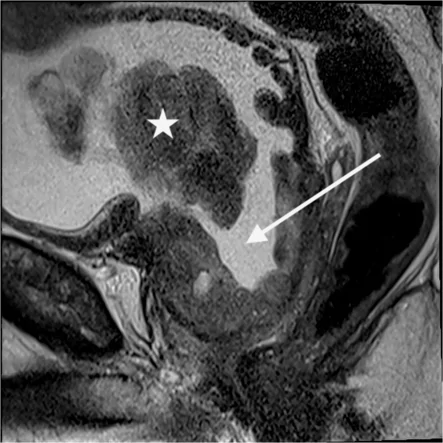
MRI Prostate performed on a different patient 6 weeks after PAE. A new prostatic defect is present at the bladder neck (white arrow). A large volume of sloughed prostatic tissue (white star) is noted within the lumen of the urinary bladder
